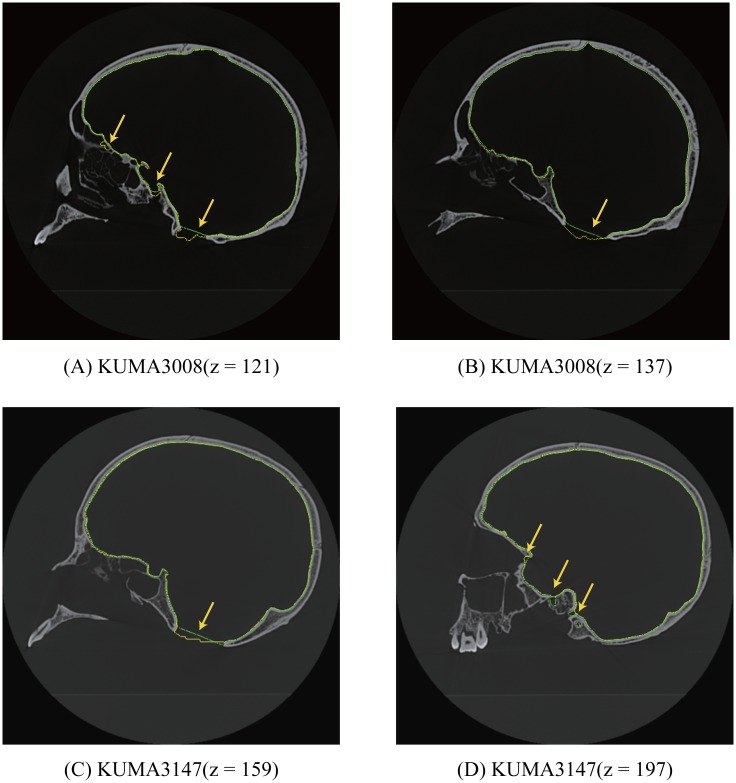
2D comparison of cross-section of CT images, polygonal models by manual operation (green) and our result (yellow). Yellow arrows show significant differences of polygons.
